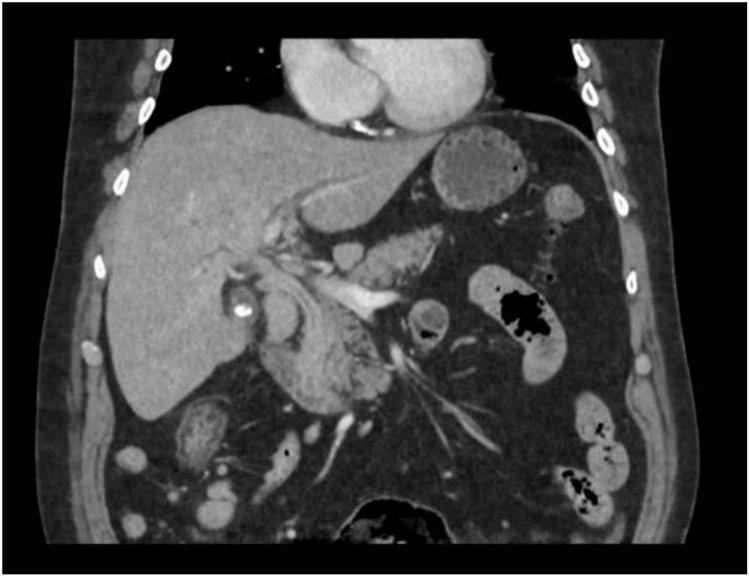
CT scan showing thickening and enhancement of the entire extrahepatic common bile duct with intrahepatic duct dilatation.
